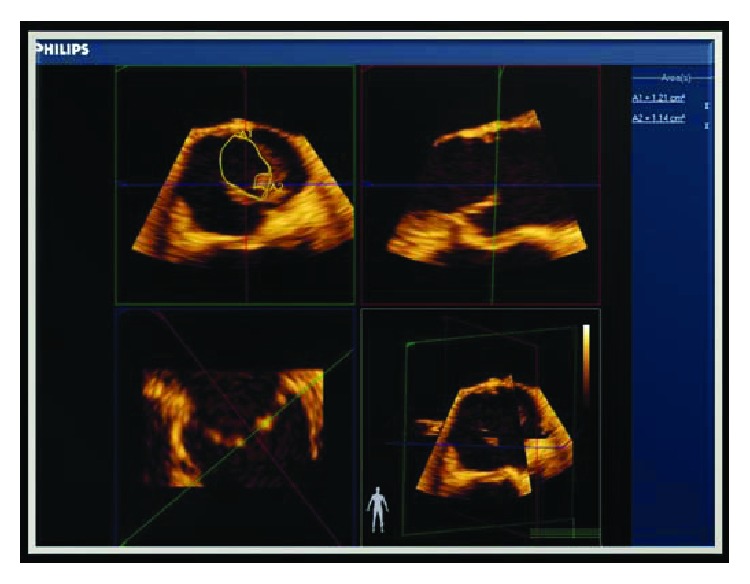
TEE 3D image (Philips Qlab) of the aortic valve using the planimetry method to measure the aortic valve area. TEE = Transesophageal echocardiography.
